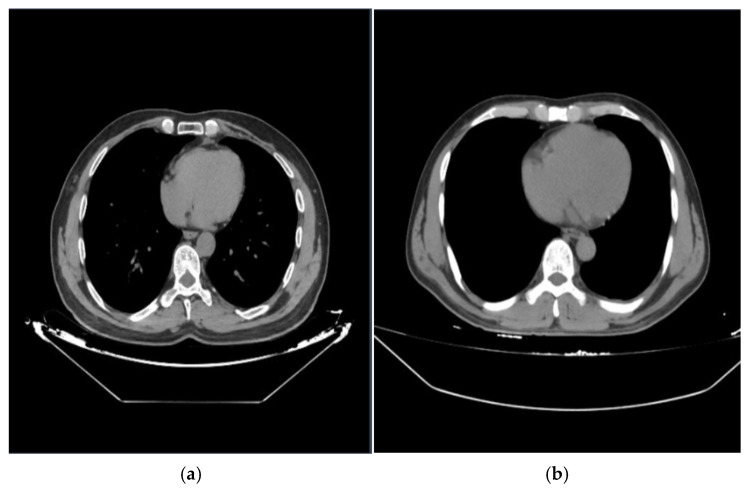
Example of a general CT image with multiple fractures: (a) random sample 1 from the dataset; (b) random sample 2 from the dataset.
